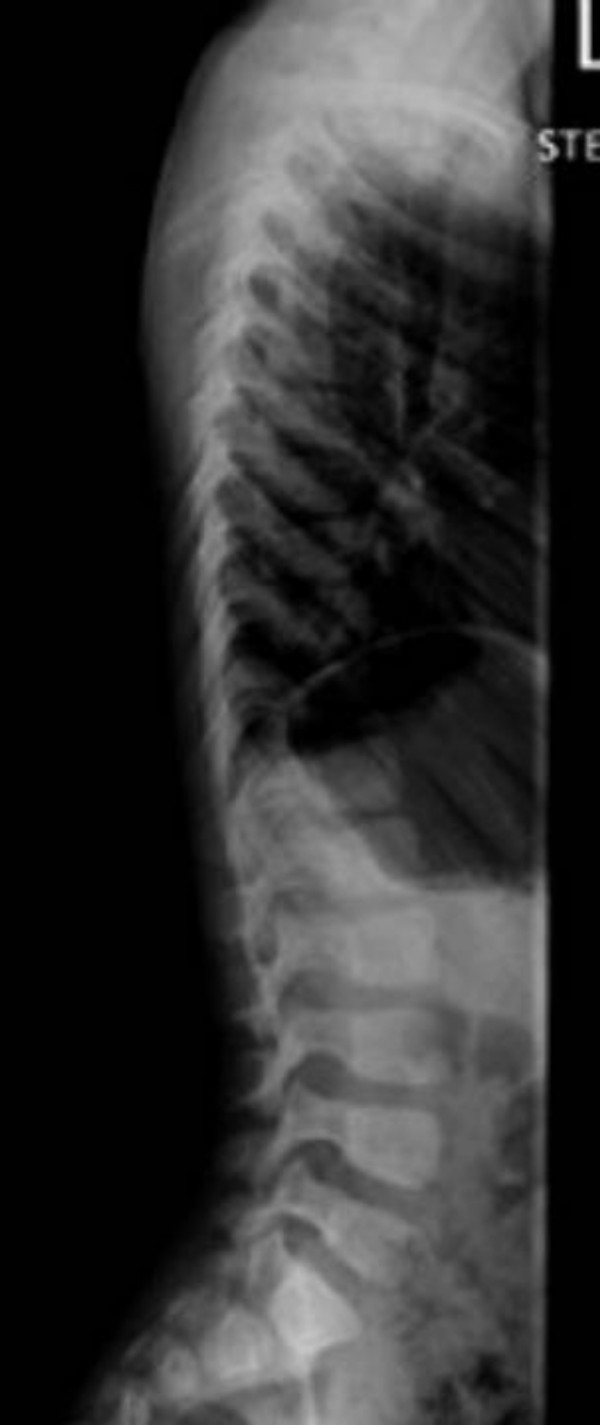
Lateral spine radiograph showed normal vertebral anatomy but with loss of the physiological lumbar lordosis.
